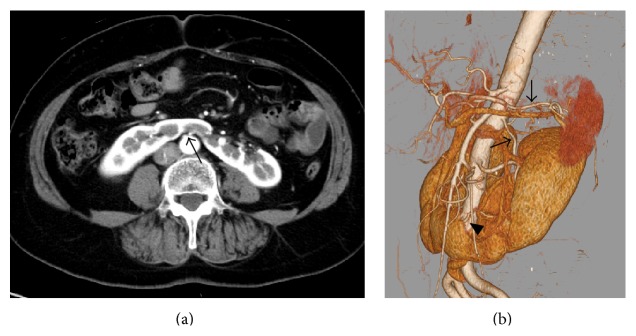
(a) Preoperative donor CT images with contrast enhancement showed the isthmus of the horseshoe kidney and an artery from the aorta supplying the isthmus (arrow). (b) Three-dimensional reconstruction of the CT scan of the donor revealed various blood vessels supplying the horseshoe kidney. Two left renal arteries (arrows) supplied the upper and middle poles of the left kidney and two aortic branches (arrowheads) supplied the isthmus.
